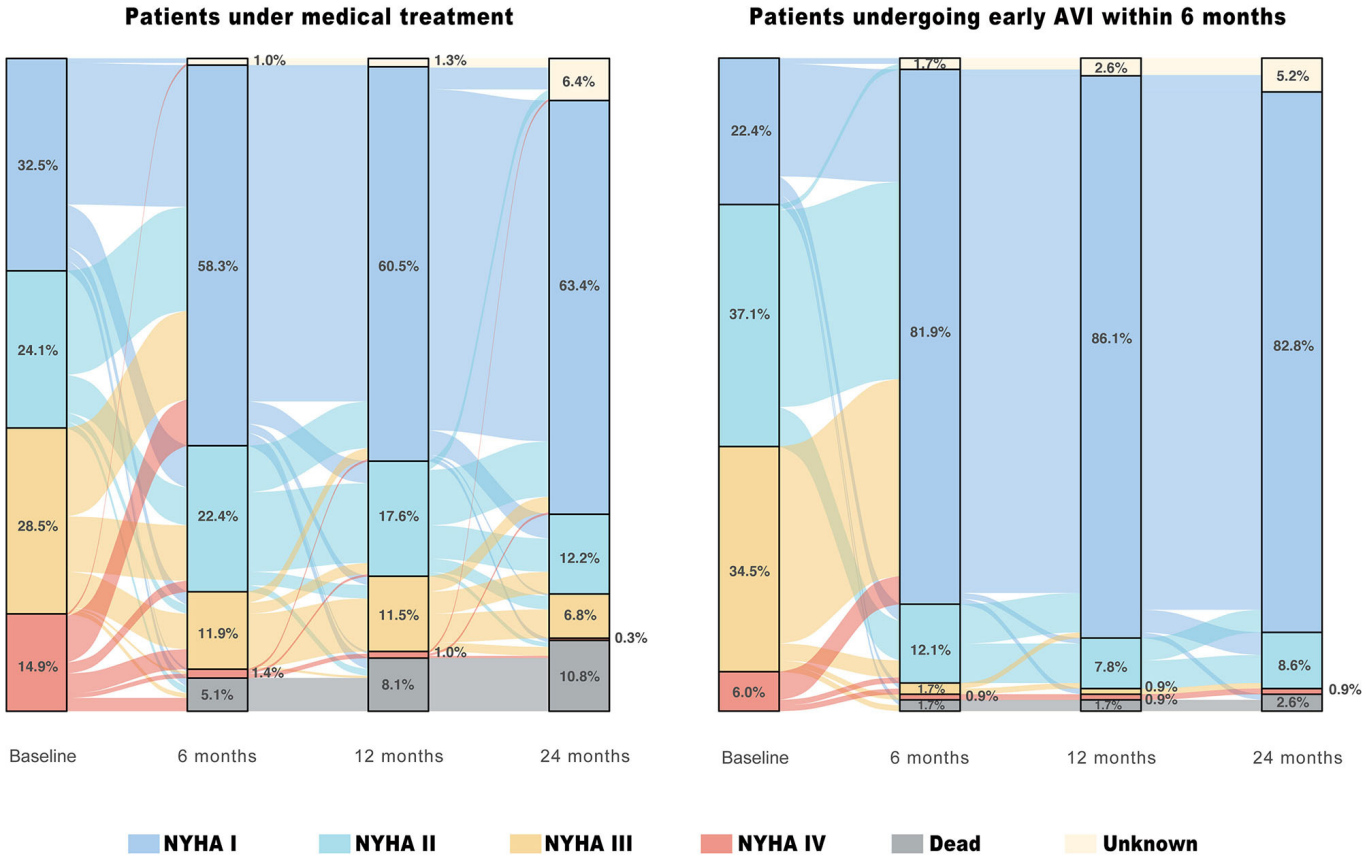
Temporal course of symptom status in patients with moderate AR and LVSD (LVEF ≤55%) under different treatment strategies. The river plots show the changes in New York Heart Association (NYHA) functional classification for patients under medical treatment who at least survived and were followed up for 15 days and for patients undergoing early aortic valve intervention (AVI) within 6 months of the baseline echocardiography.
